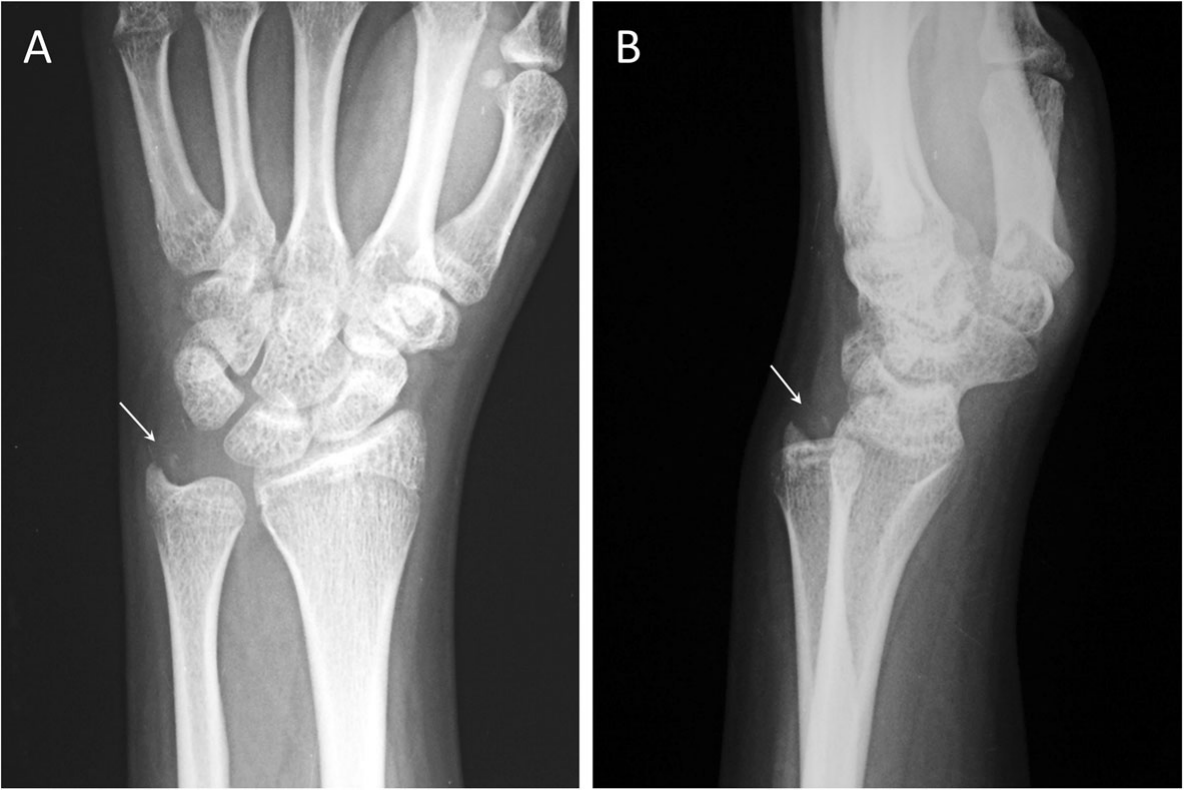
Radiographs of a 47-year-old female patients. a Posteroanterior projection showed small ulnar styloid tip fracture (arrow). b Lateral projection demonstrated diagonal ulnar side ascending course of the fracture (arrow) with subluxed distal radioulnar joint
